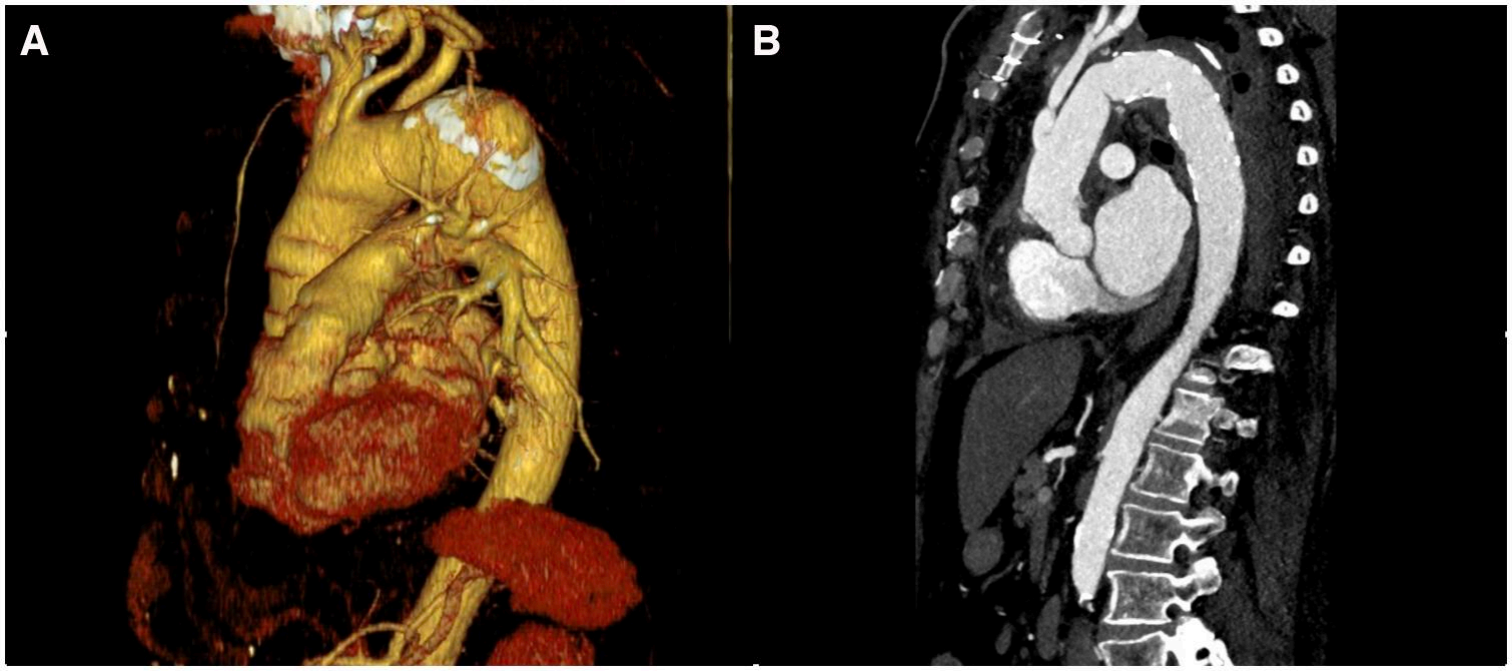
(A) Preoperative CT image. 3D reconstruction of an atherosclerotic aneurysm of the distal arch. (B) Postoperative CT image. Complete exclusion of the atherosclerotic aneurysm by the frozen elephant trunk procedure. CT: computed tomography.
